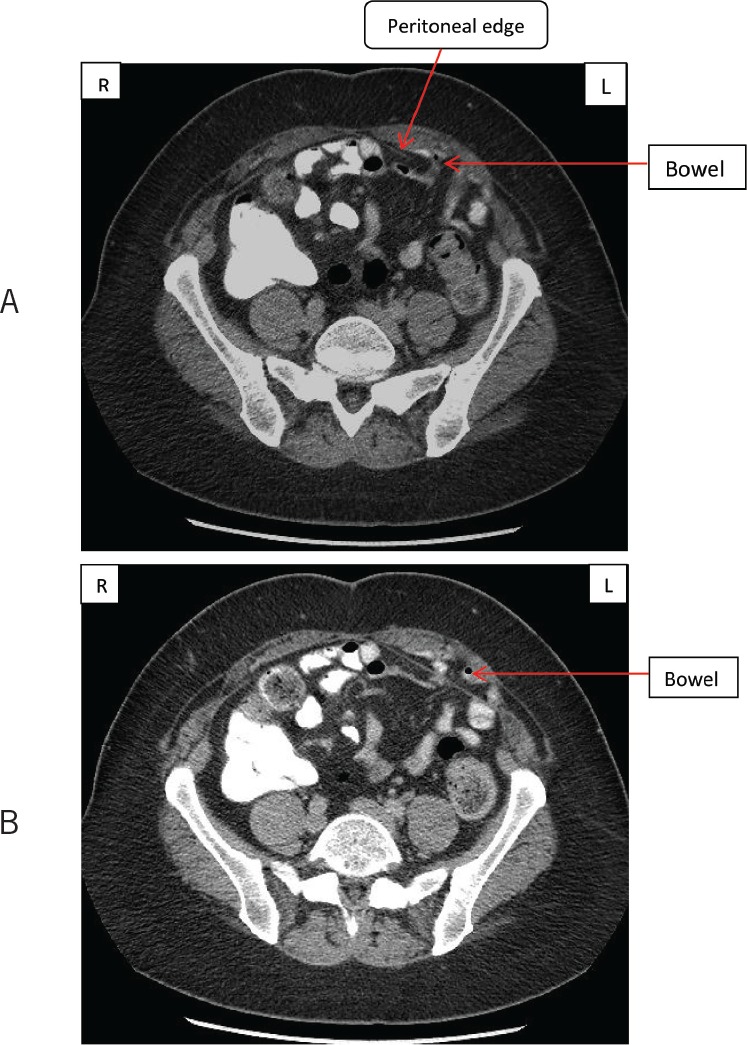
Computed tomography: the left-sided unilateral ‘peritoneal recess’ (A) and the bowel inside the peritoneal recess (arrow) (B)
